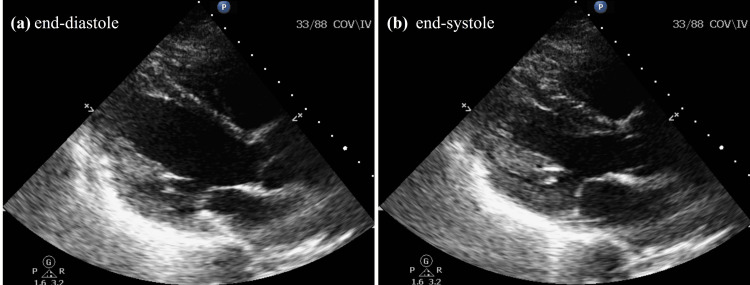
Transthoracic echocardiogram before the initiation of bicalutamide Transthoracic echocardiogram parasternal long axis view before the initiation of bicalutamide: (a) end-diastole and (b) end-systole. The left ventricular ejection fraction was 50-55% with normal left and right ventricular wall size and thickness
